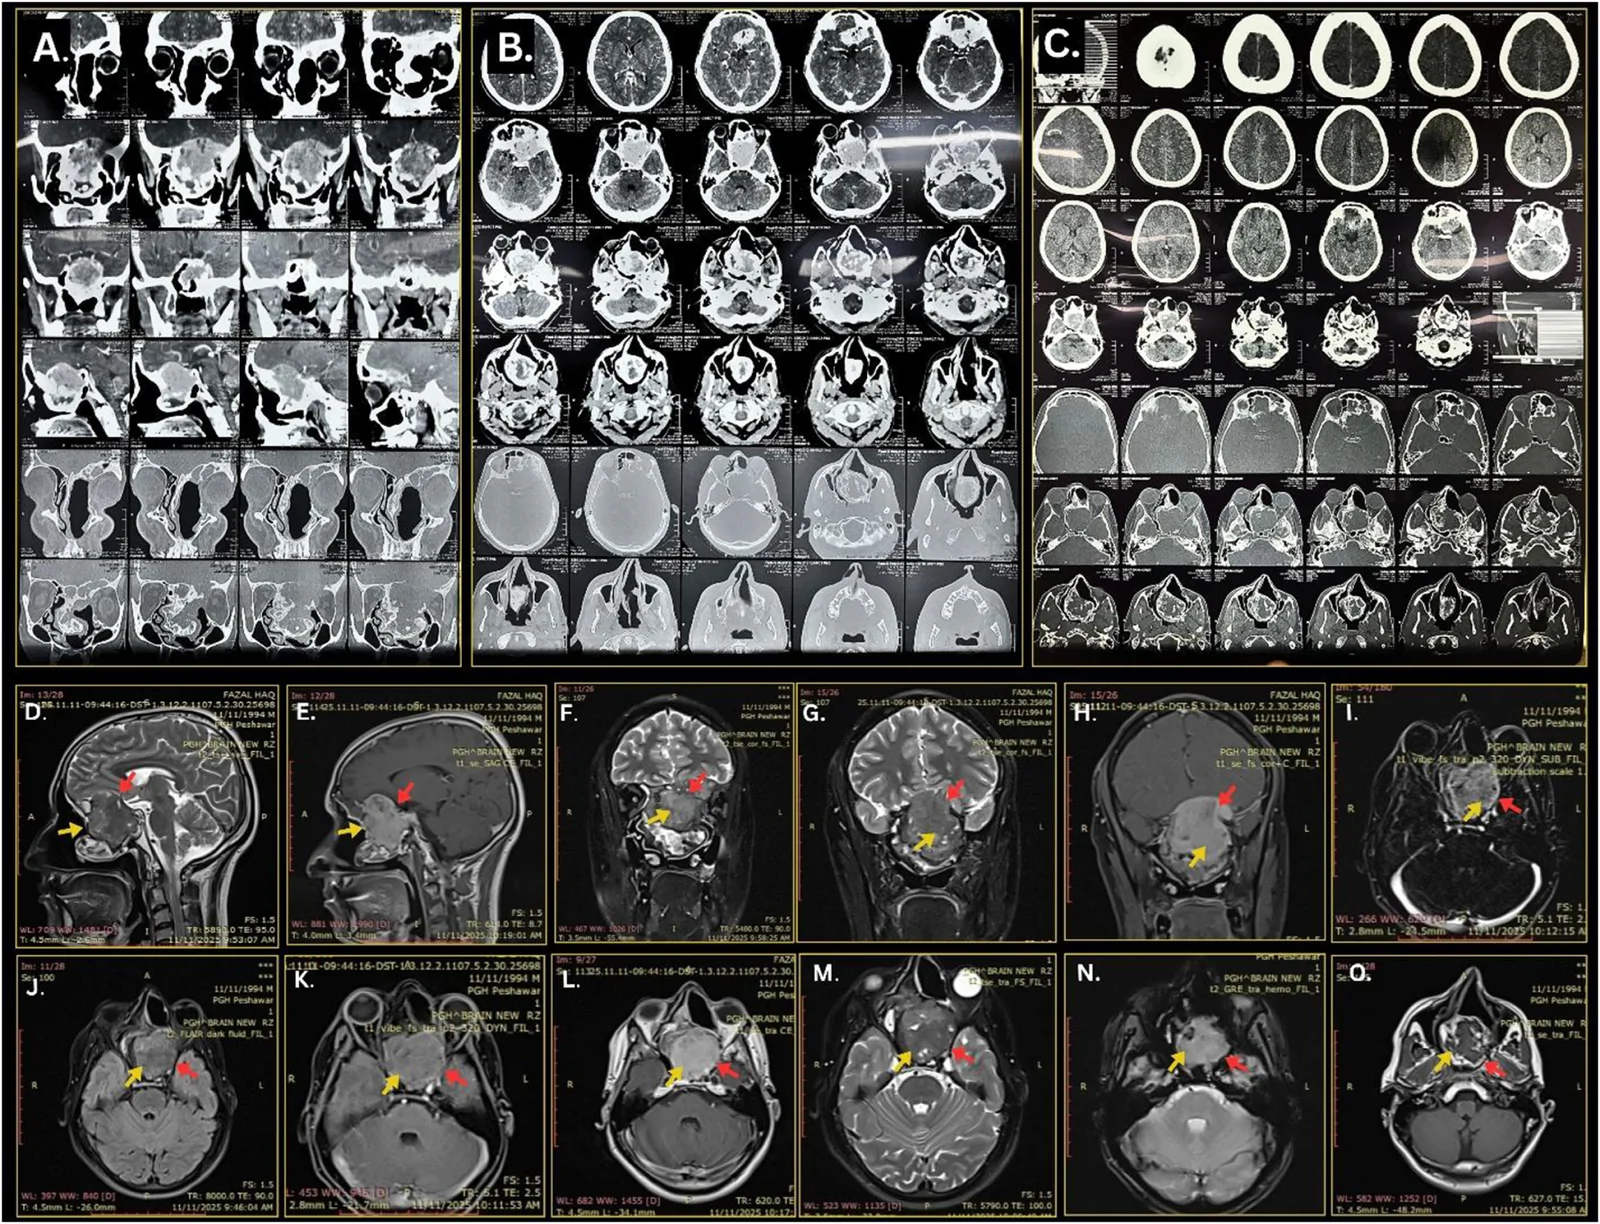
Cross-sectional imaging: (A–C) Contrast-enhanced CT (axial, coronal, sagittal) shows a large expansile, destructive lesion (5.3 × 5.3 × 5.5 cm) arising from the nasal septum and left ethmoid air cells, eroding the left lamina papyracea, extending to the left orbital apex with optic nerve compression and superiorly into the frontal bone and pituitary fossa, with epidural extension abutting the left frontal lobe. The right orbit and optic nerve are intact. (D–O) Multiplanar contrast-enhanced MRI depicts the tumor (yellow arrow), whereas the red arrows indicate the optic nerve. Imaging characterizes a 6.0 × 5.0 × 4.6 cm enhancing soft-tissue mass in the posterior left nasal cavity with marked rightward septal deviation, extending into the nasopharynx, sphenoid sinus, and sellar region with dural enhancement (meningeal tail). Critical involvement includes mass effect on the left optic nerve and abutment of the left internal carotid artery, without definitive vascular invasion.
